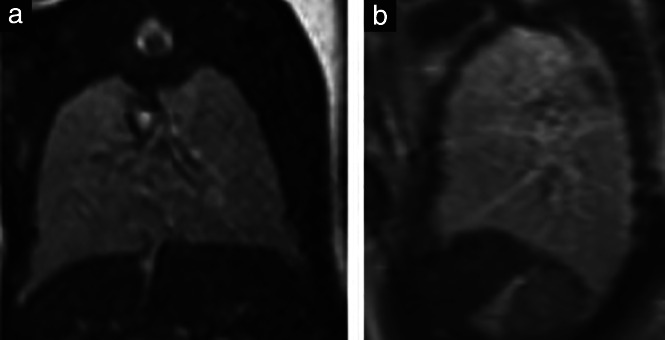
Coronal (a) and sagittal (b) T2‐weighted magnetic resonance images in a fetus with right atrial isomerism at 32 + 2 weeks' gestation. The fetal lungs had hyperintense linear signal intensity changes, with the characteristic nutmeg pattern of the lung, indicative of pulmonary lymphangiectasia, in the setting of obstructed total anomalous pulmonary venous drainage and heterotaxy.
